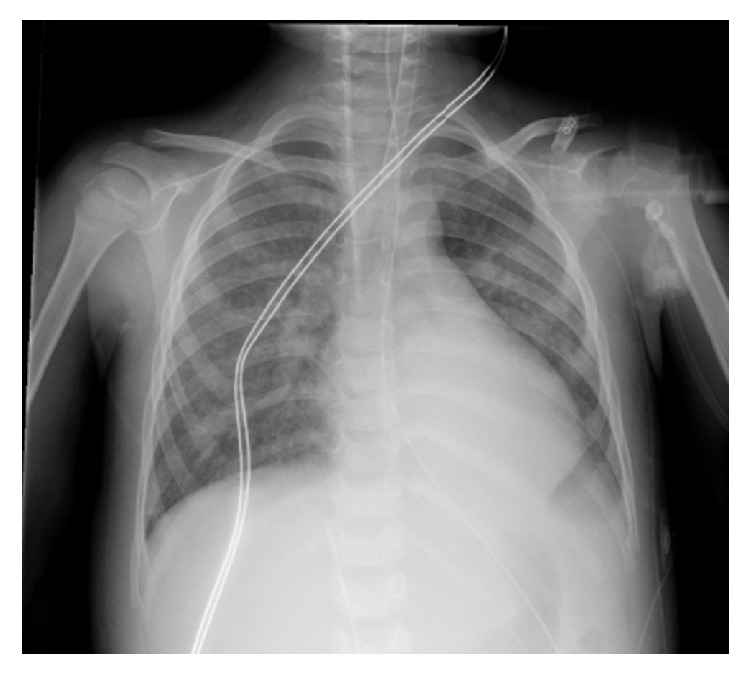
Follow-up radiograph. Improvement in pulmonary disease from previous image five days following admission and treatment. Mild perihilar consolidation persists with basal atelectasis.
